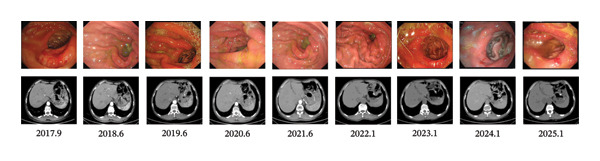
Follow‐up contrast‐enhanced CT and endoscopic images.
